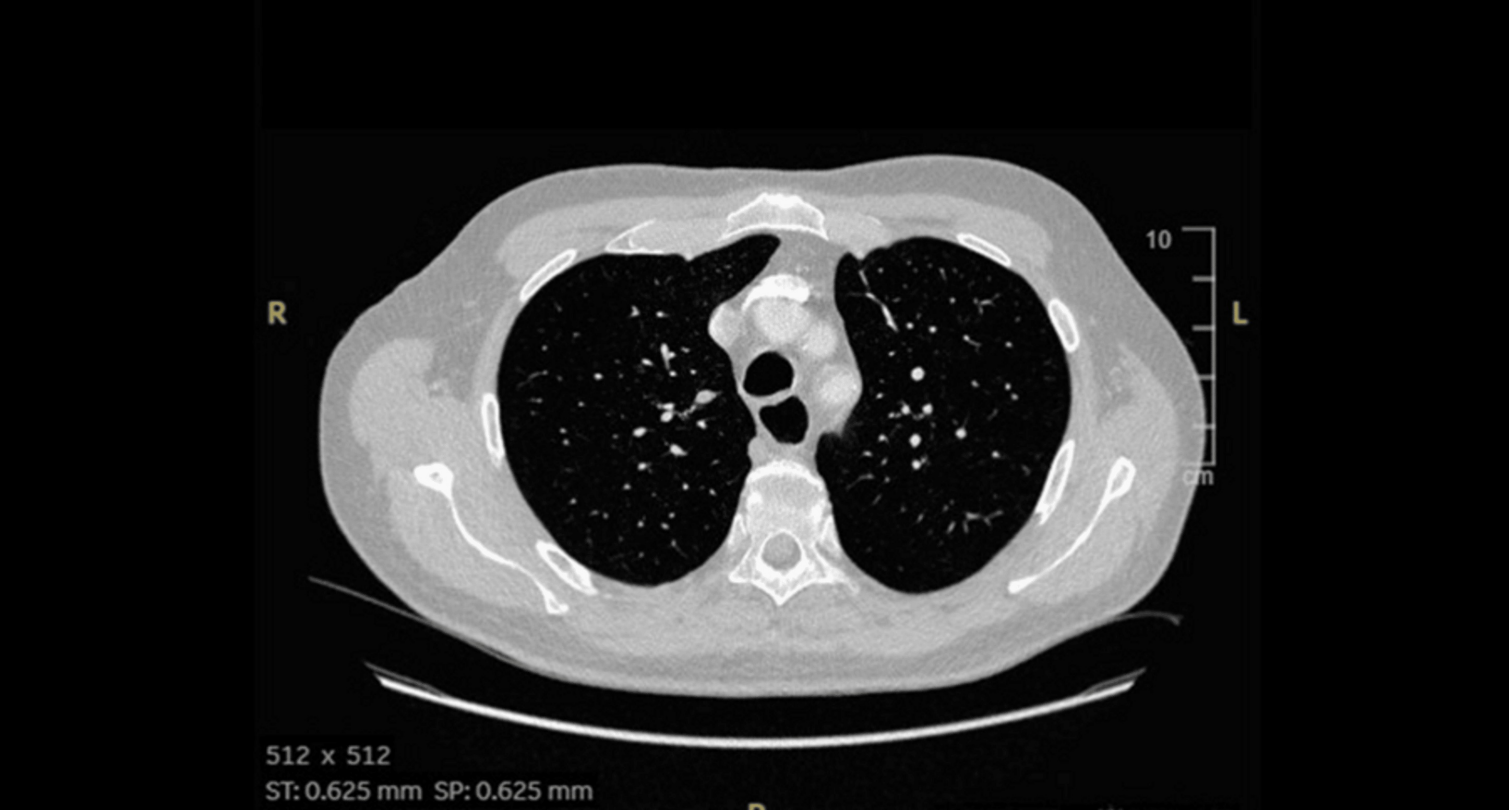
CT scan of the chest showing no thymoma CT: computed tomography
